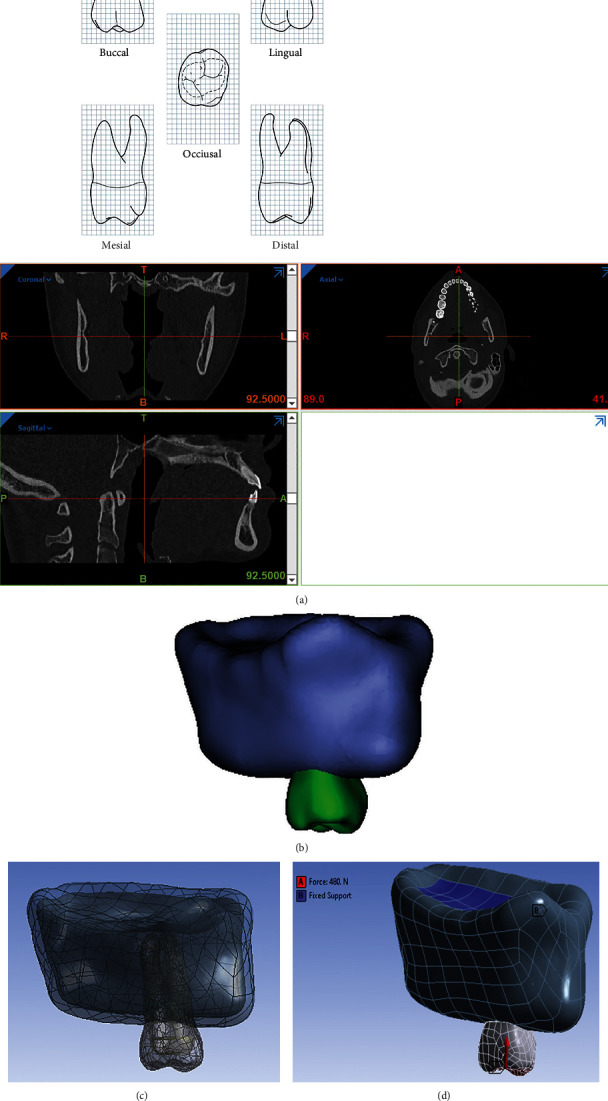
(a) Maxillary right first molar (grid = 1 sq.·mm) CT scan images. (b) Model in STL format. (c) Model in STP format. (d) Application of vertical and oblique forces.
